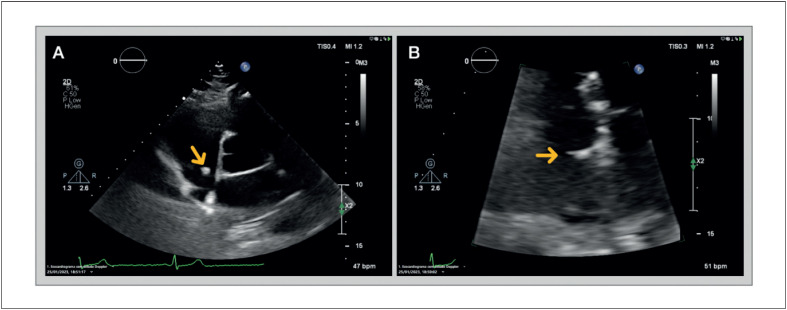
Ecocardiograma transtorácico PSAX ao nível da valva aórtica (A) e apical 4 câmaras (B; ampliado), evidenciando massa arredondada na aurícula direita, ecogênica, móvel, de etiologia duvidosa, com cerca de 6 mm de diâmetro, em possível relação com a válvula de Eustáquio.
